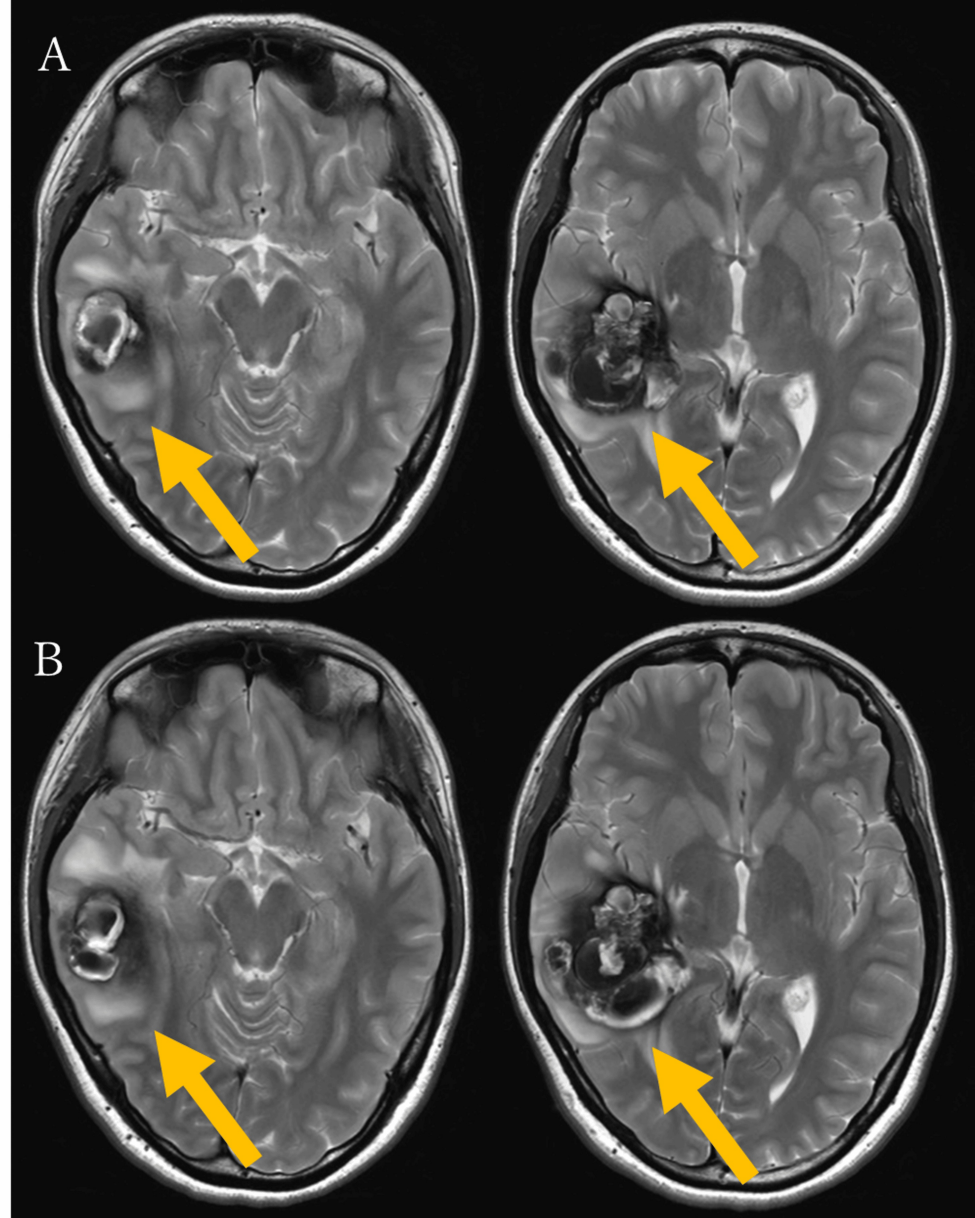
Follow-up brain MRI for the lesion (A) Brain MRI on hospital day 18 (17 days after initial presentation) shows rebleeding and lesion enlargement with a maximum diameter of 37.5 mm on T2-weighted imaging. (B) Brain MRI on day 32 from initial presentation reveals a second rebleeding event and further enlargement of the lesion to 46.8 mm on T2-weighted imaging.
